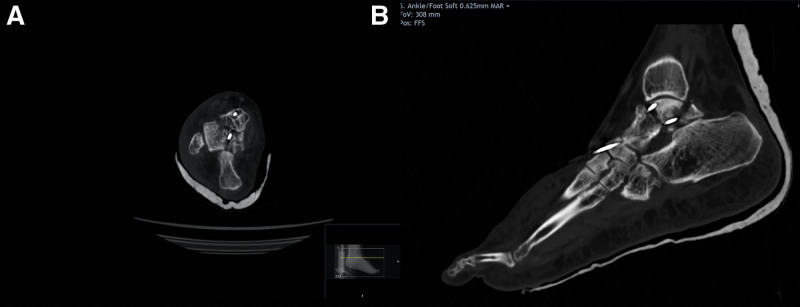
CT scan of the ankle. (A) Axial and (B) sagittal cuts delineating the fracture lines. CT = computed tomography.
